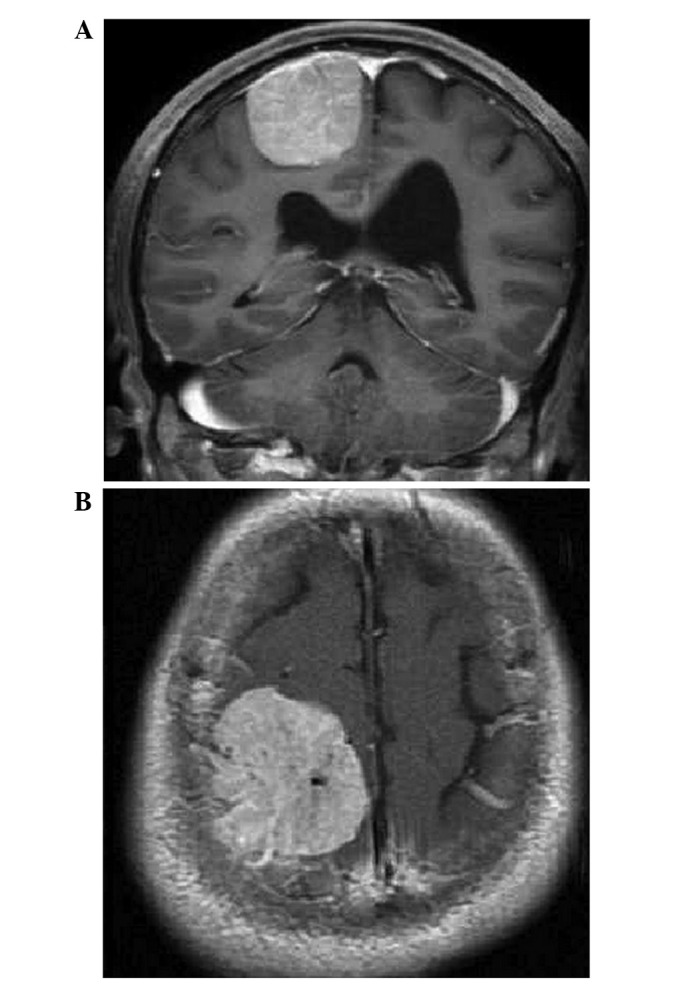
Pre-operative magnetic resonance imaging (MRI) of parasagittal meningioma in the central gyrus region. (A) coronal position; (B) horizontal position.
